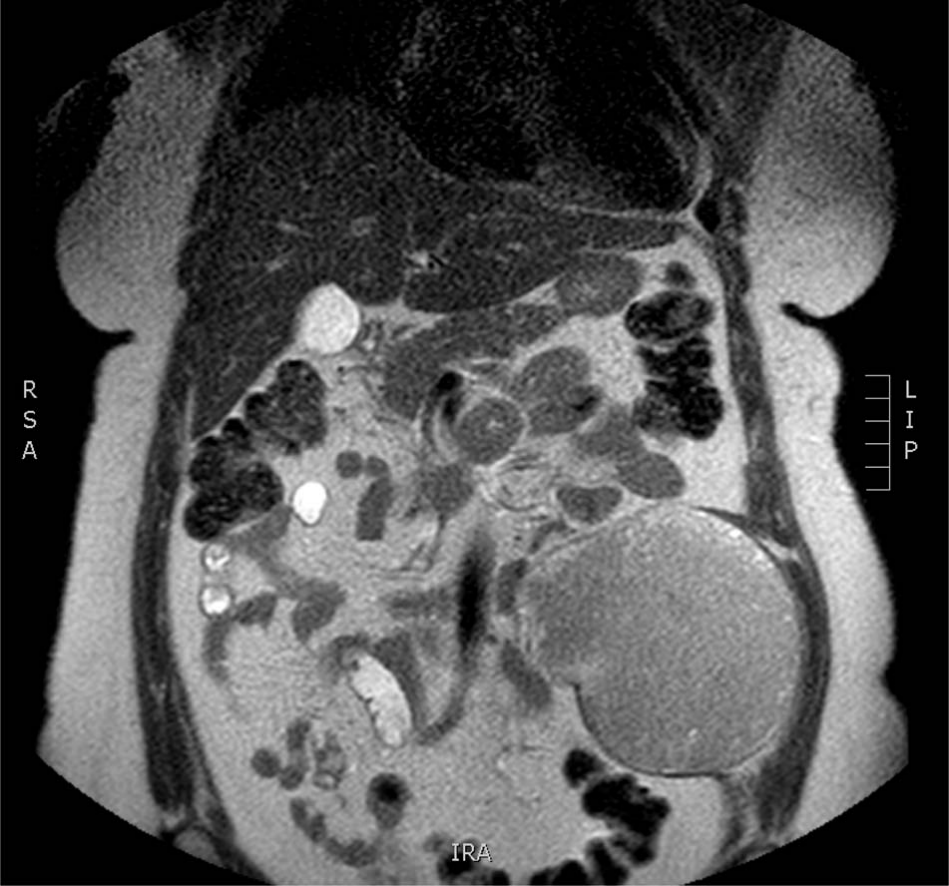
Preoperative MRI—axial plane. T1 weighted image. High sign density of the lesion.
